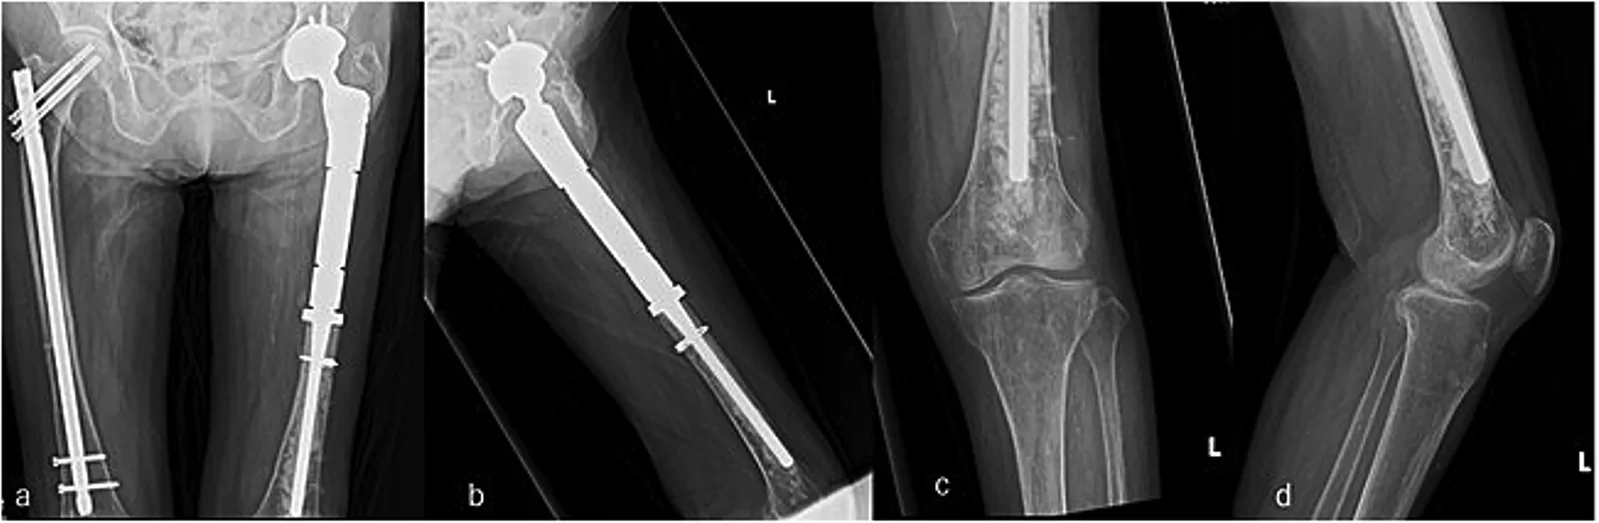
Plain radiographs at six months postoperatively (a–d). No evidence of implant loosening or new fracture is identified.
